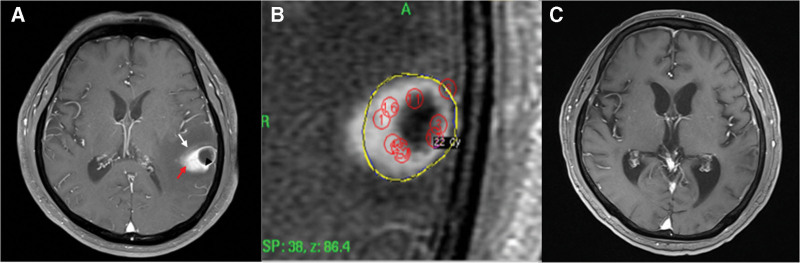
A representative GKRS-case1 for brain metastasis with contrast media leakage. (A) Contrast-enhanced brain MRI reveals a 3.4-cm heterogeneously-enhancing mass with contrast media leakage on the left temporal lobe (tumor: arrowhead, contrast media leakage within edema: red arrow). (B) GKRS was performed on the tumor with a marginal prescription dose of 22 Gy at 50% prescription isodose, deliberately avoiding the distant regions of contrast media leakage. (C) The enhancing mass had achieved complete remission on brain MRI. GKRS = gamma knife radiosurgery, MRI = magnetic resonance imaging.
